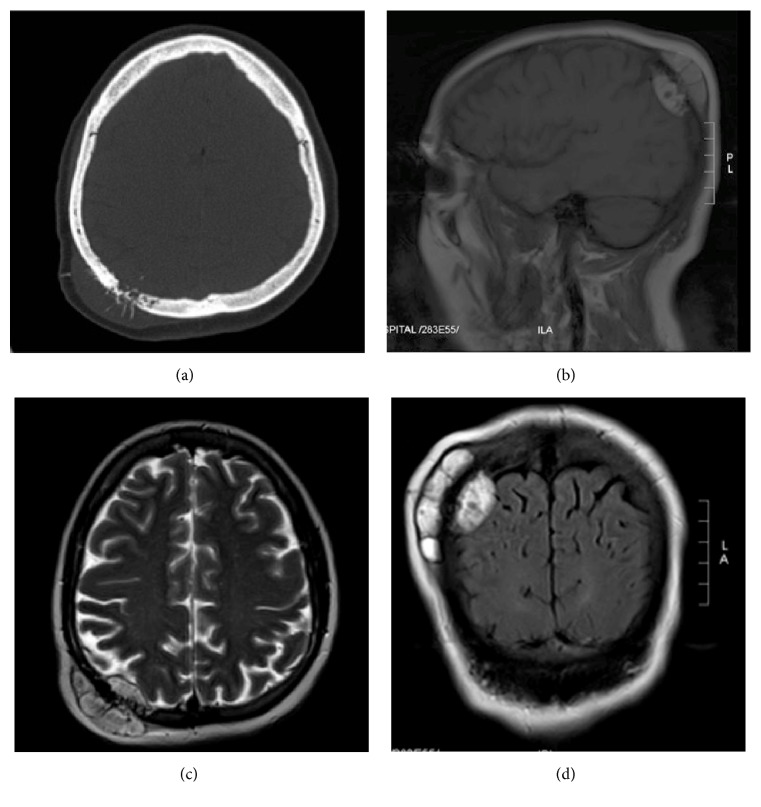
(a) Preoperative axial CT scan of the brain (bone window) showing osteolytic changes with associated soft tissue swelling. (b) Preoperative unenhanced parasagittal T1-weighted MRI image showing hyperintense epidural cystic mass compressing the cortex underlying. (c) Preoperative axial T2-weighted MRI image showing mildly hyperintense mass on the right parietal region. (d) Preoperative FLAIR coronal MRI showing hyperintense mass that traverses the skull.
